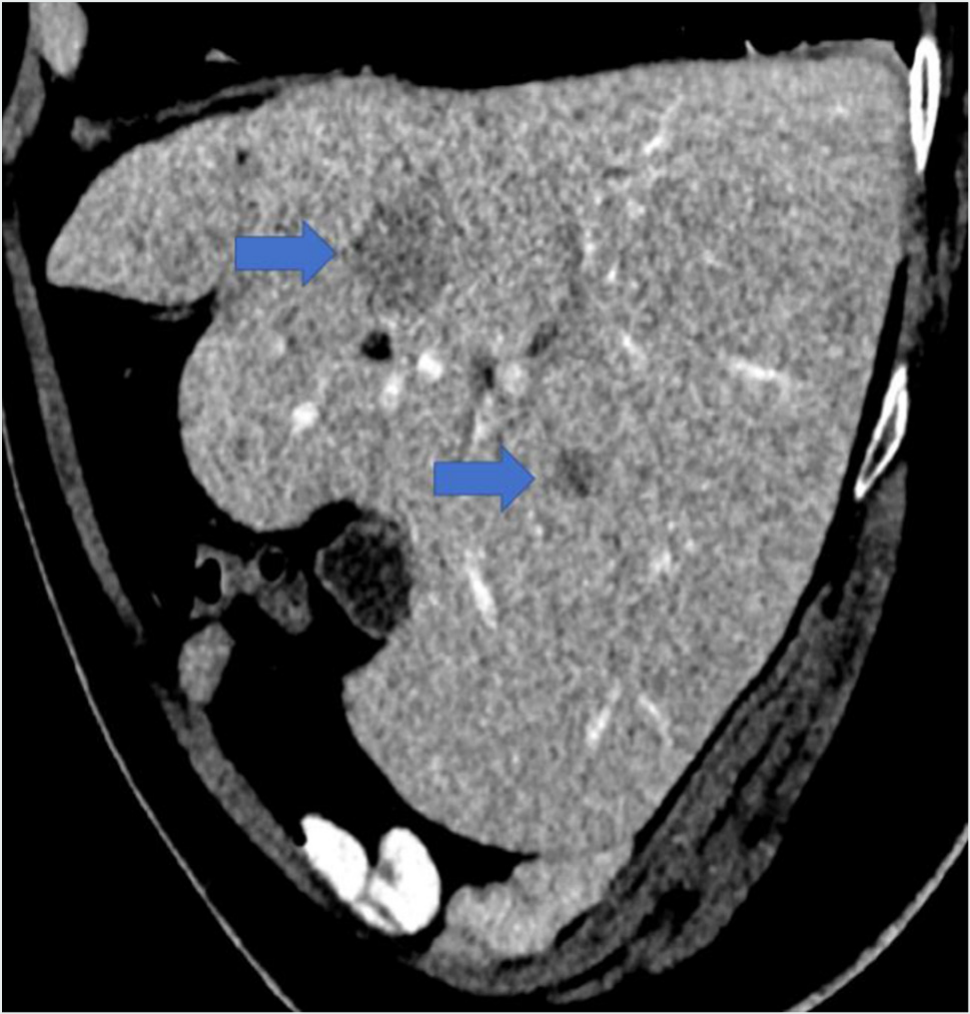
Ultrasound (US) of the hepato-biliary tract confirmed two homogeneous strongly hypoechoic liver nodules, measuring 20 and 12 mm in diameter. The lesions’ margins were well-defined, with neither hypoechoic halo sign, nor posterior acoustic enhancement or shadowing (arrow, Figure 2).
